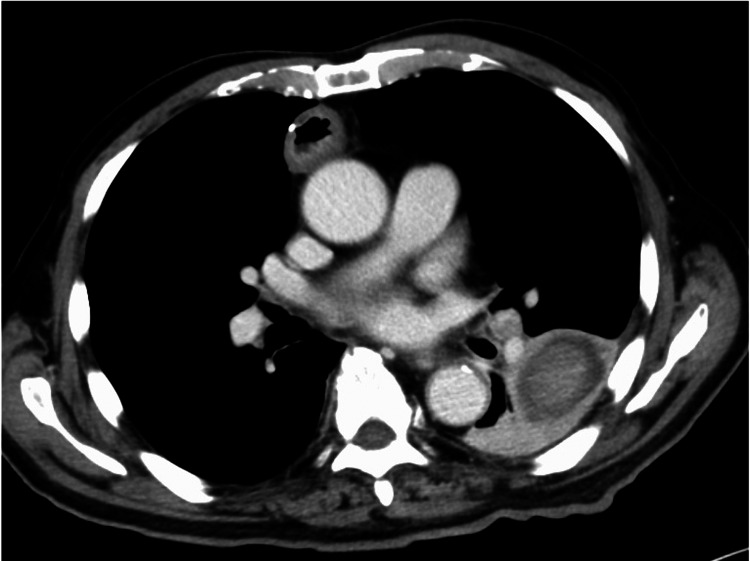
Follow-up chest contrast-enhanced CT reveals a reduction in the abscess size and no enhancement of the PAP. PAP: Pulmonary artery pseudoaneurysm
